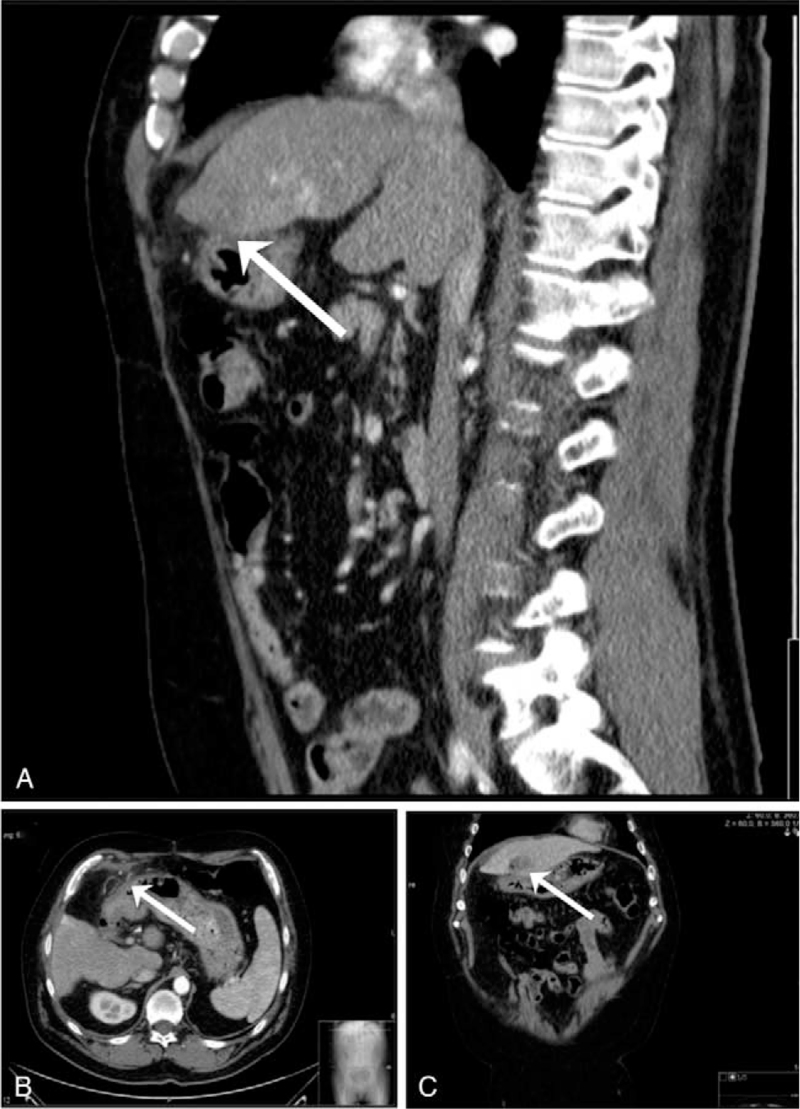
Contrast enhanced computed tomography 6 weeks after percutaneous microwave ablation of hepatocellular carcinoma nodules in segments III and VIII. Sagittal and horizontal images reveal the integrity of the gastric wall in the area of the former perforation (A, B, arrows). Frontal images show the intact gastric wall in direct vicinity to the necrotic ablation area (C, arrow).
